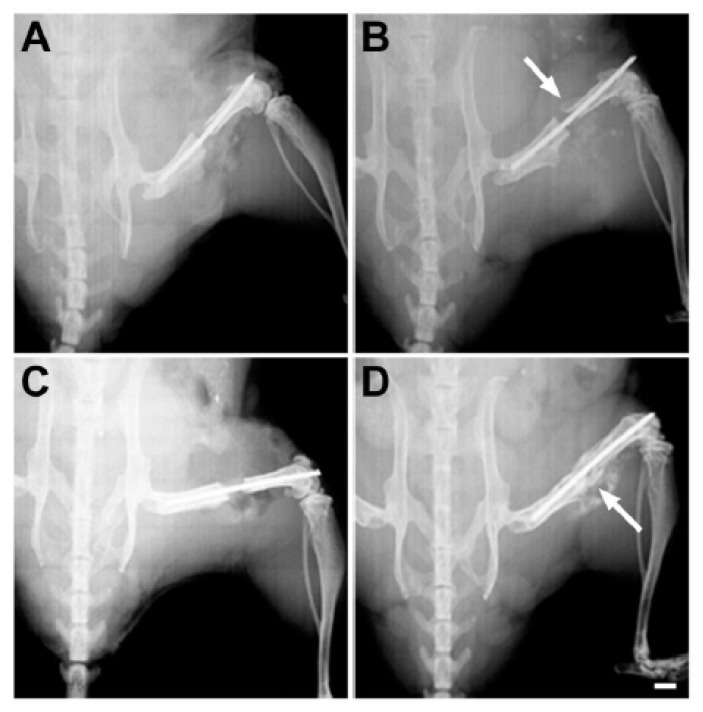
Bone formation in response to therapy was monitored by in vivo X-ray examination of the segmental defect. (A) The defect with the scaffold/BMSC control at surgery. (B) A small hard callus was often visible in defects with this control, as seen on the distal side of the defect at 8 weeks post-surgery (arrow), but bone formation had not proceeded beyond this stage. (C) The scaffold in the scaffold/BMSC/SAG21k-IOX2 therapy was often easily visible at surgery. (D) Mineralized tissue was obvious in the therapy defect at 8 weeks post-surgery (arrow). Scale bar = 1 mm.
